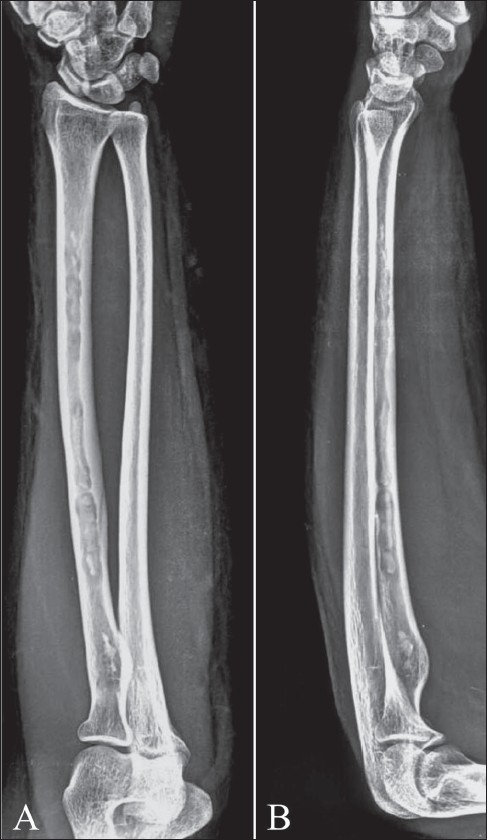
Chronic tuberculous osteomyelitis of the radius in an adult patient. Frontal (A) and lateral (B) radiographs of the left forearm show multiple areas of bone destruction with intralesional sequestration in the diaphysis of the radius. Note also diffuse involucrum formation
